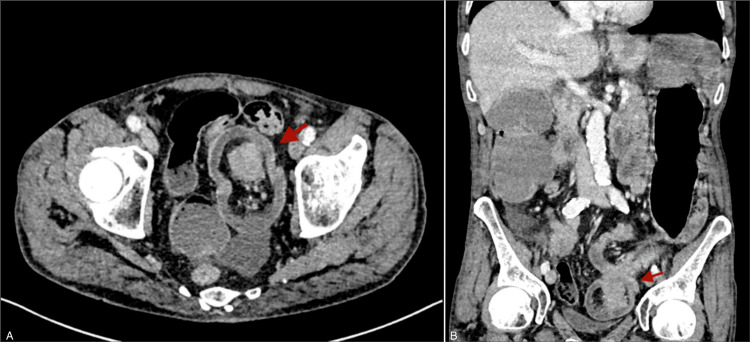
CT with intravenous contrast of the abdomen (A) Axial computed tomography (CT) scan (portal venous phase) of the abdomen shows colon wall enlargement, fat within the intussusception, and a lesion within the lumen of the colon (red arrow). (B) Coronal computed tomography (CT) scan (portal venous phase) of the abdomen shows the same finding as (A) with colo-colic intussusception.
